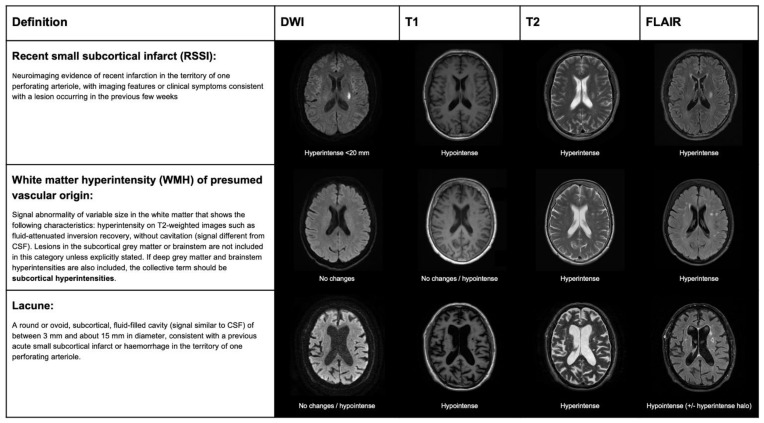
STandards for ReportIng Vascular changes on nEuroimaging (STRIVE) classification for ischemic lesions on MRI, produced by lacunar stroke.
